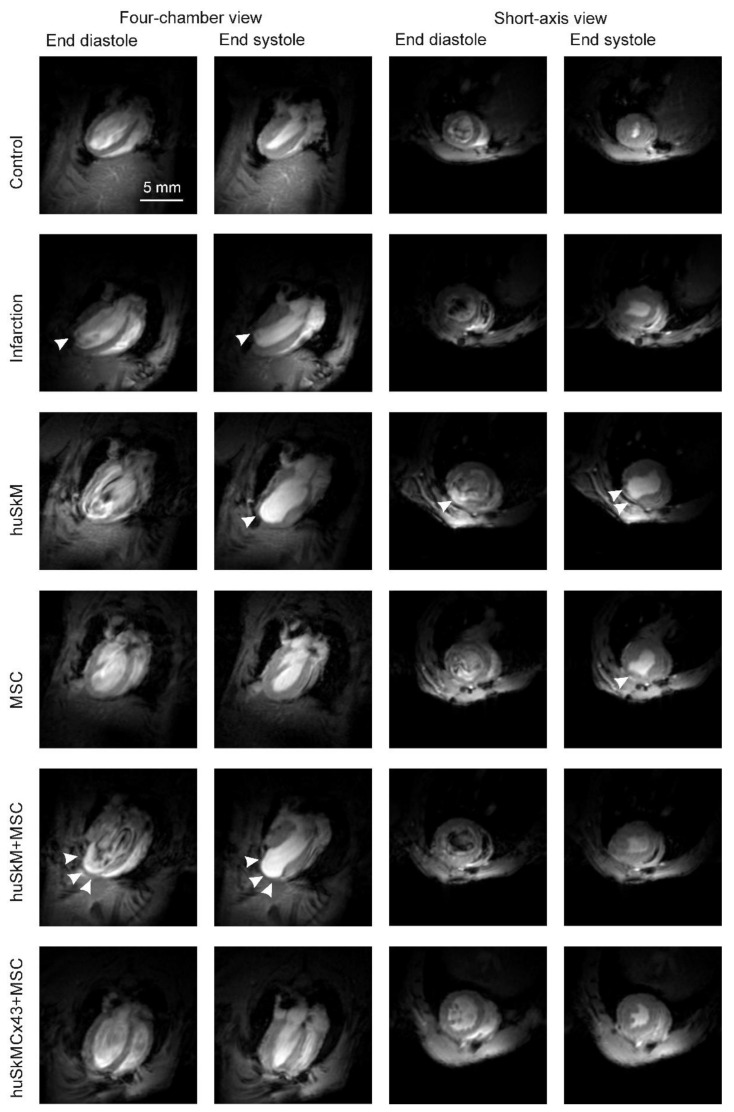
Representative MR images of control hearts (no interventions), post-infarction hearts, and healthy hearts after huSkM, MSCs, huSkM + MSC or huSkMCx43 + MSC cell interventions. The first two columns represent the 4-chamber view (end-diastole is the first column and end-systole is the second column); the next two columns represent the short-axis view (end-diastole is the third column and end-systole is the fourth column). The infarct area is indicated by arrows.
